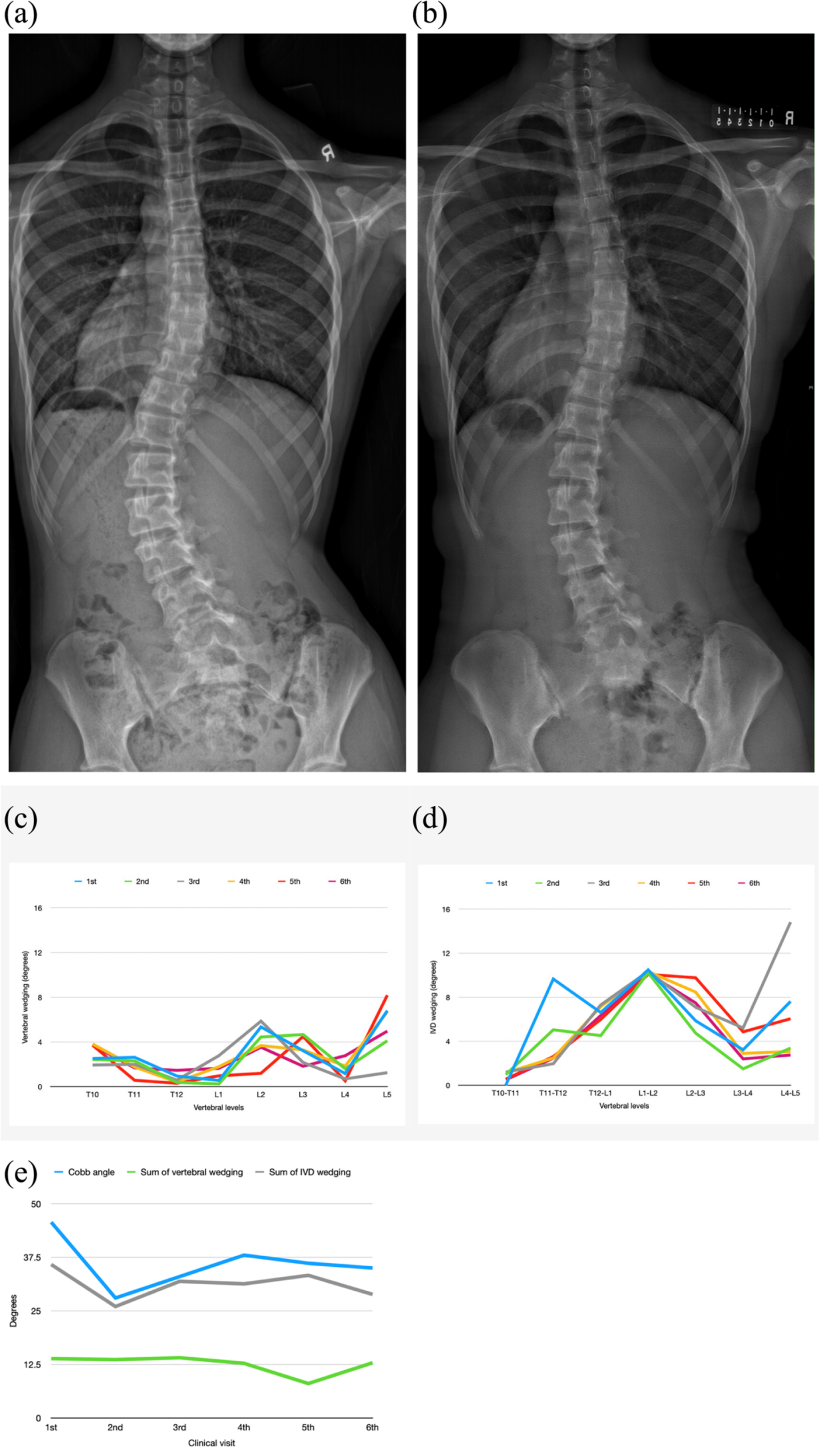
Non-progressed curves. a X-ray at the first clinical visit. T4/T11 = 25.2°. T11/L4 = 45.7°. b X-ray of the latest clinical visit. T4/T11 = 26°. T11/L4 = 35°. c Vertebral wedging of the curve T11/L4. The wedging at the apex (L2) increased by 0.5° at the 3rd clinical visit. d IVD wedging of the curve T11/L4. The wedging at the apex (L1/L2) was maintained at around 10°. e Summation of the wedging value of the lumbar curve. The sum of vertebral wedging decreased from 13.9° to 12.9° and that of IVD wedging decreased from 35.8° to 28.8°
